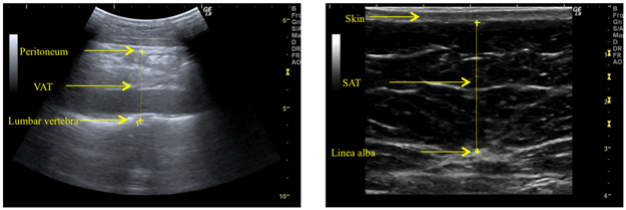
Examples of ultrasound images of VAT and SAT.
